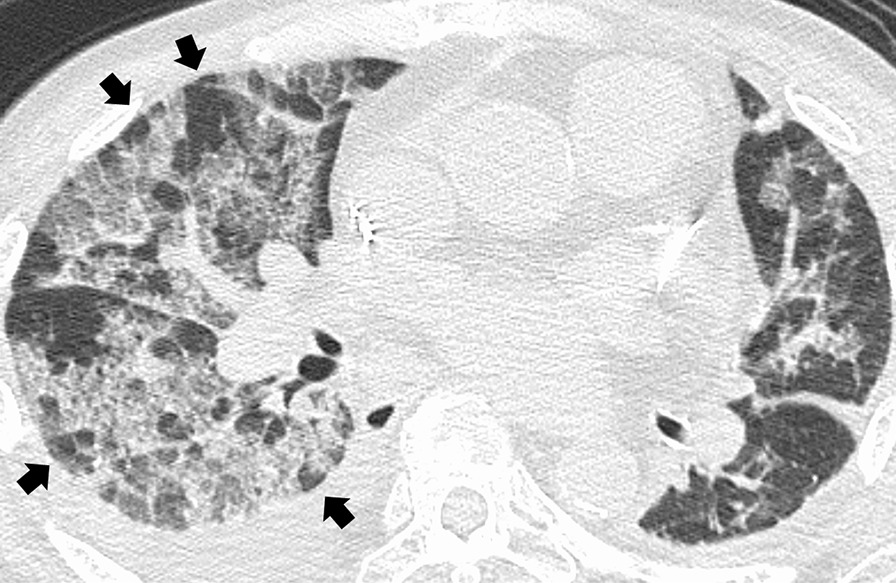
A 70-year-old man, who developed respiratory failure during antibiotic therapy for *Staphylococcus aureus* bacteremia, was diagnosed with acute respiratory distress syndrome. High-resolution CT at diagnosis showed bilateral diffuse reticulation with geographic appearance (black arrows), well-circumscribed infiltrations partially spared in secondary pulmonary lobule units, and bilateral pleural effusions. Although the patient was placed on mechanical ventilation in addition to being administered antibiotics, he passed away 4 days after diagnosis, and autopsy was performed. Pathological findings of the lungs revealed hyaline membrane formation along dilated alveolar ducts and alveoli, with shrinkage of adjacent alveoli and hyperplasia of type II pneumocytes, representing the exudate phase of diffuse alveolar damage
